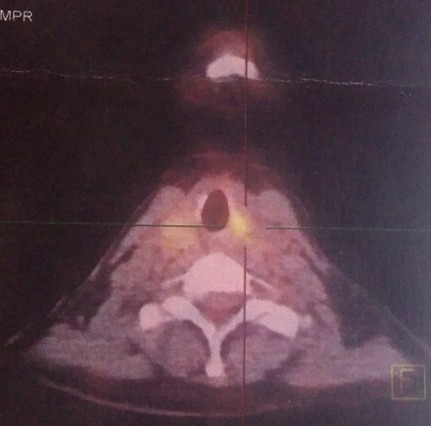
Foyer hyper métabolique de la loge thyroïdienne postérieur gauche (SUV max 3,59) compatible avec une récidive ou une maladie néoplasique résiduelle
